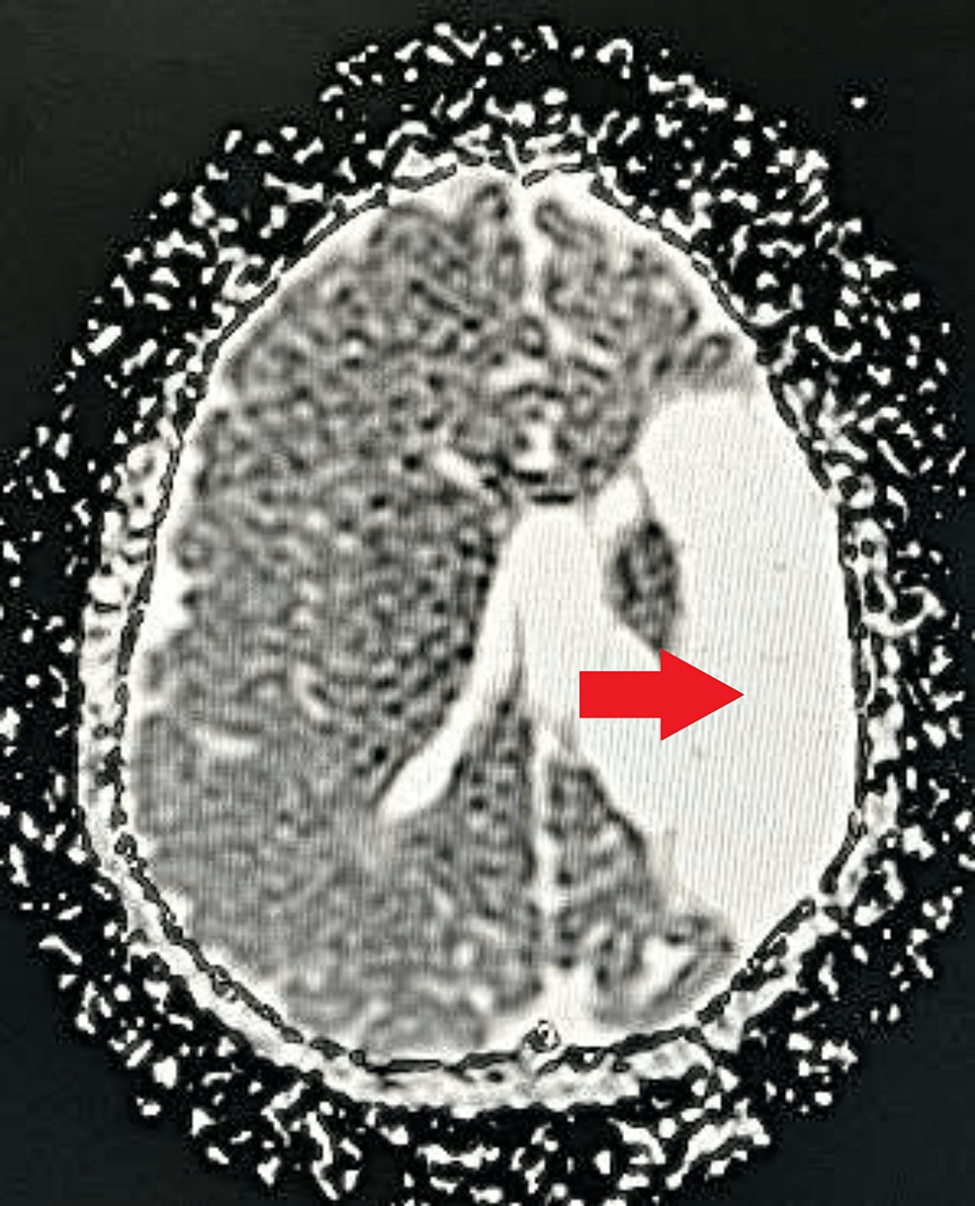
MRI brain axial ADC image Evidence of high ADC value is noted at the frontoparietal lobe on the left side Red arrow: high ADC value at the left frontoparietal lobe ADC: apparent diffusion coefficient; MRI: magnetic resonance imaging
